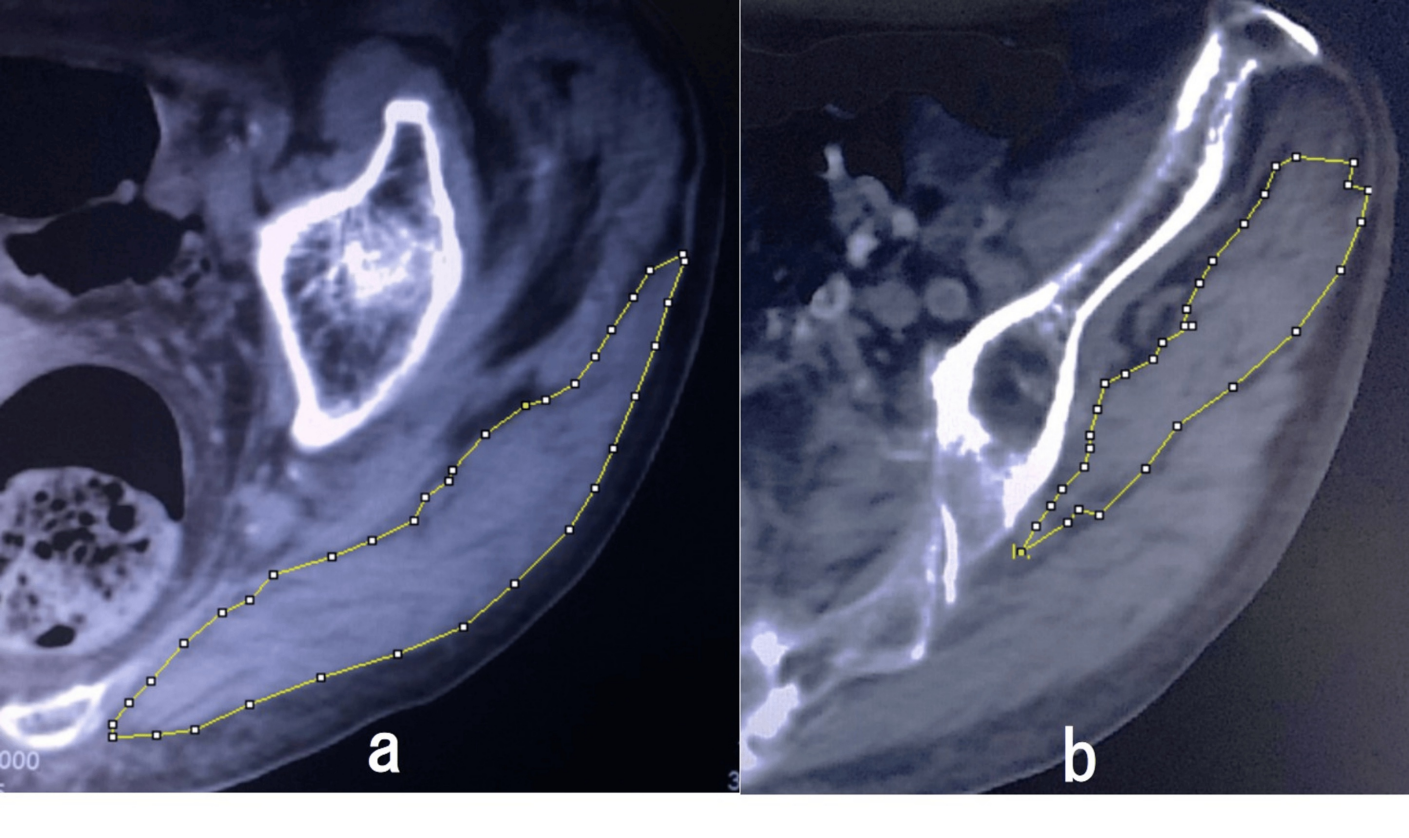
CT images of the gluteus maximus and medius muscles Panel (a) shows an axial image at the level of the greater trochanter's tip, highlighting the gluteus maximus. Panel (b) displays an axial image at the level of the third sacral vertebra, representing the gluteus medius.
